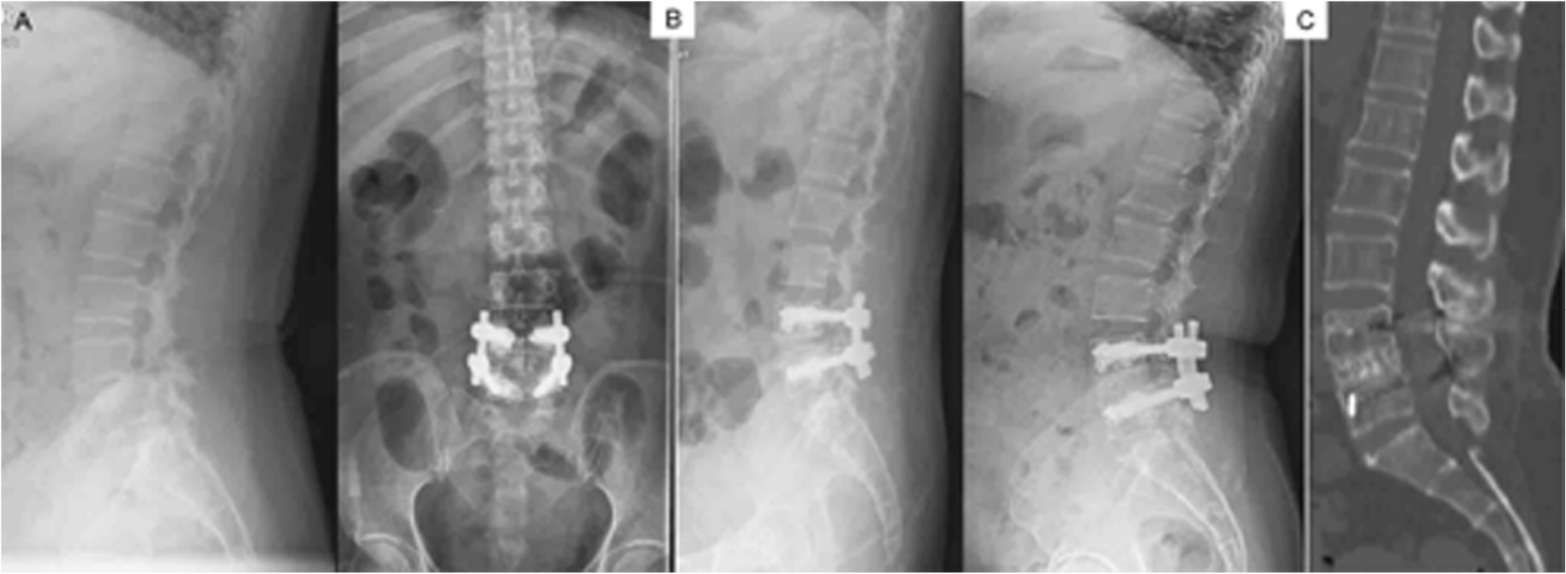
X-ray and CT images. (A) before; (B) immediately after surgery; and (C) at the final follow-up at 57 months in a 67-year-old woman with a 2-year history of lower back pain. L4 spondylolisthesis and severe osteoporosis was diagnosed (T-score=–3.7). The patient underwent transforaminal lumbar interbody fusion with PMMA-augmented CICPSs bilaterally, and the displacement was completely corrected. No screw loosening occurred, and successful fusion was achieved at the final follow-up of 57 months. Low back pain was ameliorated.
